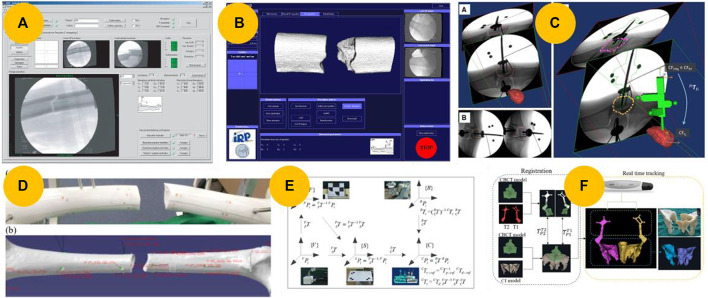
Intraoperative registration and navigation technologies. (A) User interface for a navigation system based on 2D fluoroscopy (Westphal et al., 2006). (B) User interface for navigations based on 3D fluoroscopy (Westphal et al., 2008). (C) An image registration framework enables the registration of pre-operative CT images and intra-operative fluoroscopic images of a fractured bone using a custom-made fiducial marker (Dagnino et al., 2017b; Dagnino et al., 2017c). (D) Twenty-four fiducial markers attached to a femur mockup for paired-point matching (Lee et al., 2013). (E) Transformations of coordinate systems among the model, robot, surgical operation, and visual coordinate systems (Li et al., 2015b). (F) Real-time 3D navigation enabled by registering the cone beam computed tomography (CBCT) and CT models (Shi et al., 2021).
